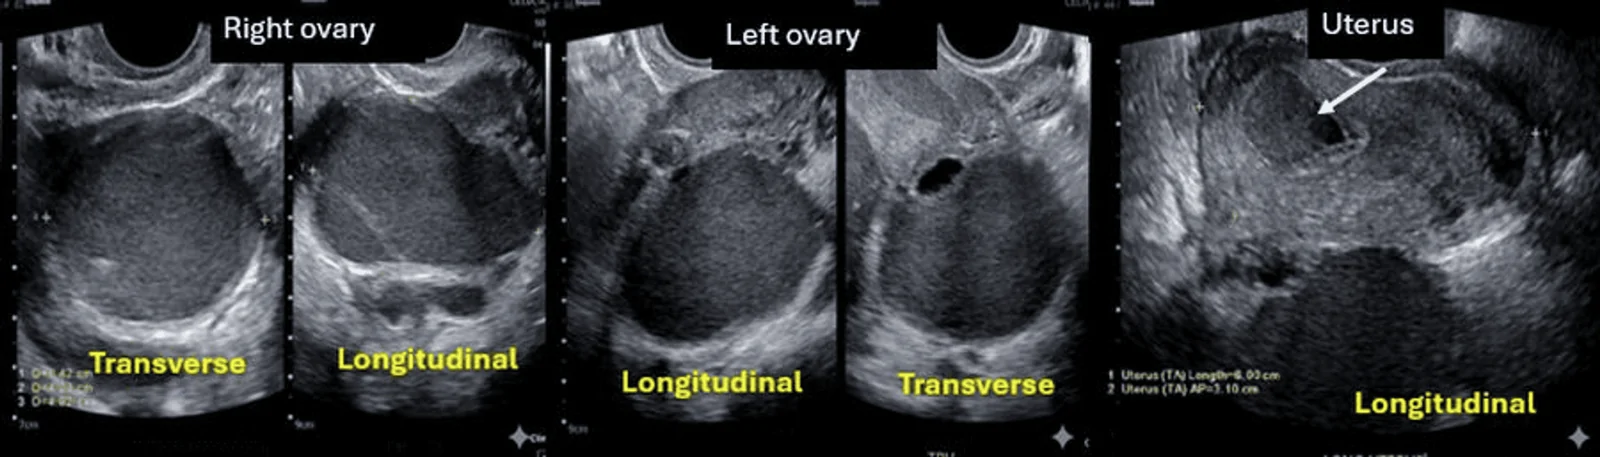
Follow-up TVUS of the adnexa and endometrial cavity Transverse and longitudinal views of the right and left adnexa demonstrate no interval change in the bilateral complex ovarian cysts, which most likely represent endometriomas. Less benign cystic lesions cannot be excluded. A probable dilated fallopian tube adjacent to the right complex cyst may also contain blood products. No hyperemia is observed to suggest an abscess, and there is no evidence of ovarian torsion. The uterus is visualized. Complex fluid distends the fundal endometrial cavity (white arrow), possibly representing blood products. TVUS: transvaginal ultrasound
